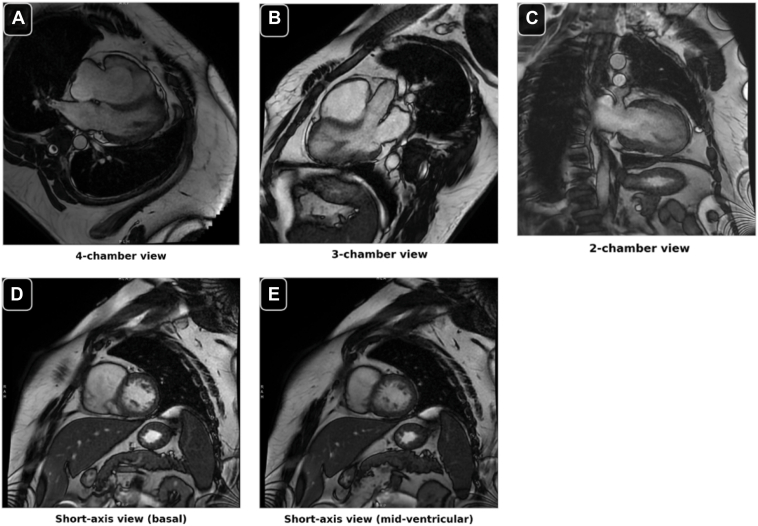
Cardiac magnetic resonance imaging demonstrating left ventricular noncompaction (LVNC). (A) 4-chamber view, (B) 3-chamber view, (C) 2-chamber view, and (D and E) short-axis views at basal and mid-ventricular levels. Prominent trabeculations are seen involving the mid-ventricle to apex, with a noncompacted-to-compacted myocardial ratio exceeding 2.3/1 at end-diastole, fulfilling the Petersen cardiac magnetic resonance diagnostic criterion for LVNC.
